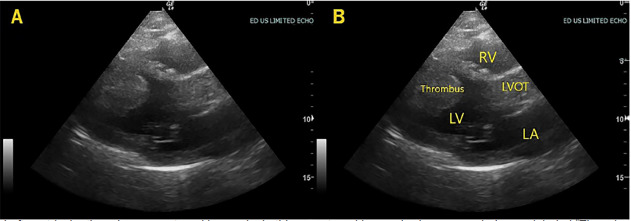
Left ventricular thrombus, parasternal long axis. In this parasternal long-axis view, a rounded mass labeled “Thrombus” in frame B is seen in the apex of the left ventricle. *RV*, right ventricle; *LVOT*, left ventricular outflow tract; *LA*, left atrium, *LV*, left ventricle.
